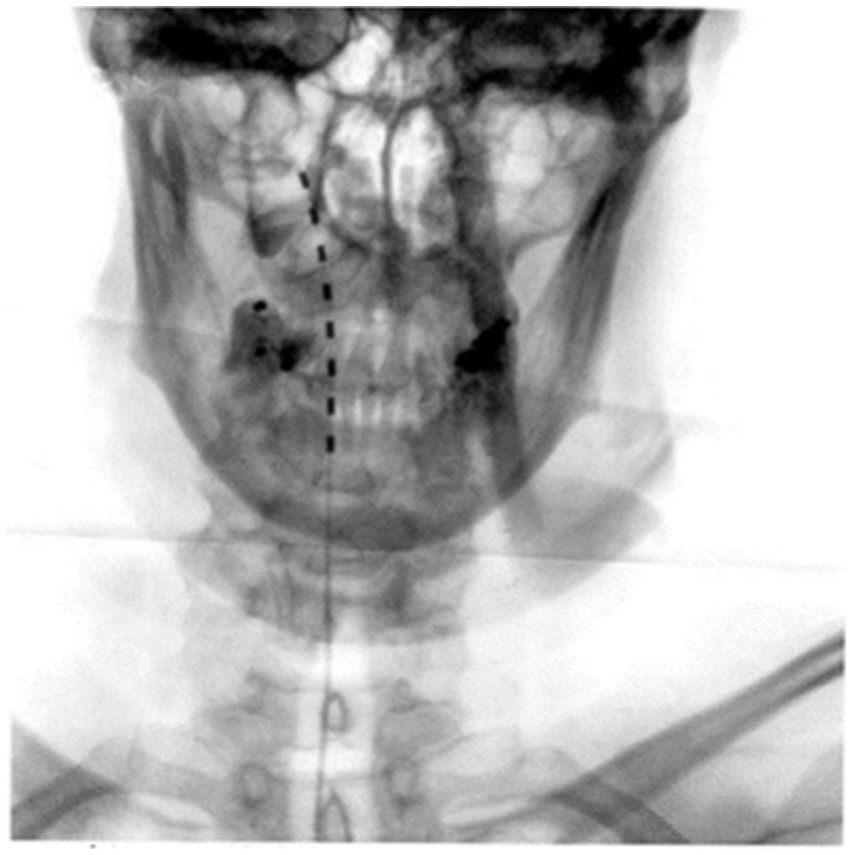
Radiographic image showing the correct placement of the epidural 8-contact lead at the cervicomedullary junction (C1–C2 level) in the anteroposterior view.
